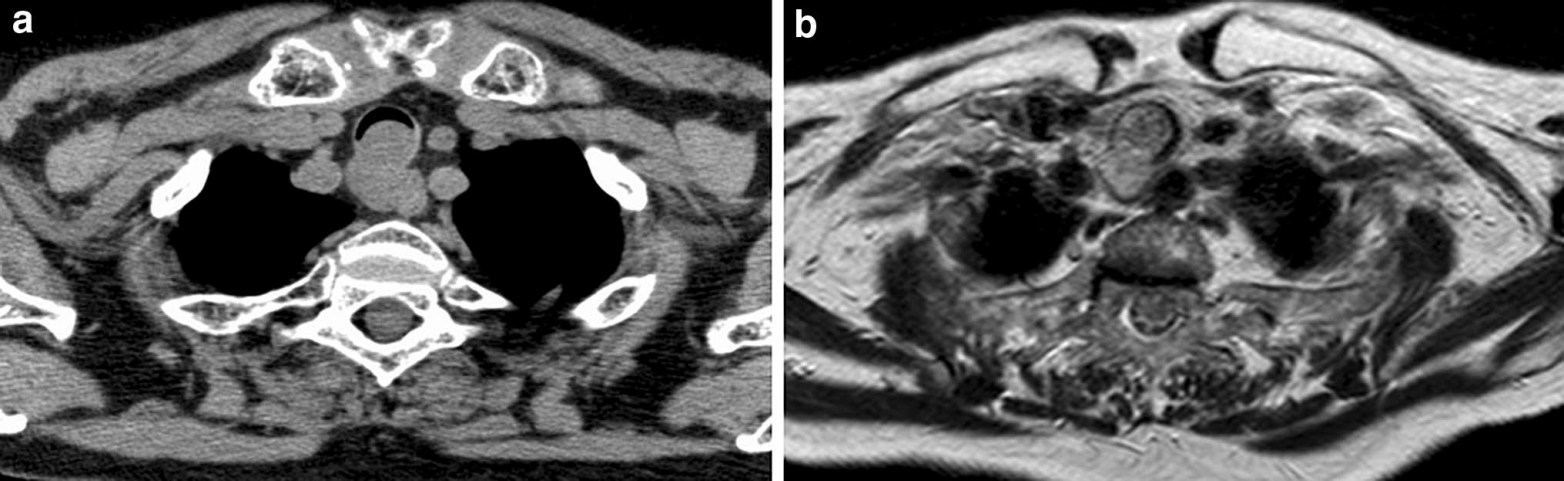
Computed tomography (CT) and magnetic resonance imaging (MRI) findings revealed a 1.5 cm large border and a smooth surface nodule shadow on the posterior wall of the thoracic trachea approximately 5 cm below the glottis. Mass formation with a major axis of 1.0 cm in diameter extending from the trachea to the esophagus was observed. Based on MRI findings, the border with the surrounding organs was clear, and it was diagnosed as primary tracheal tumor invasion (CT: a, MRI: b)
